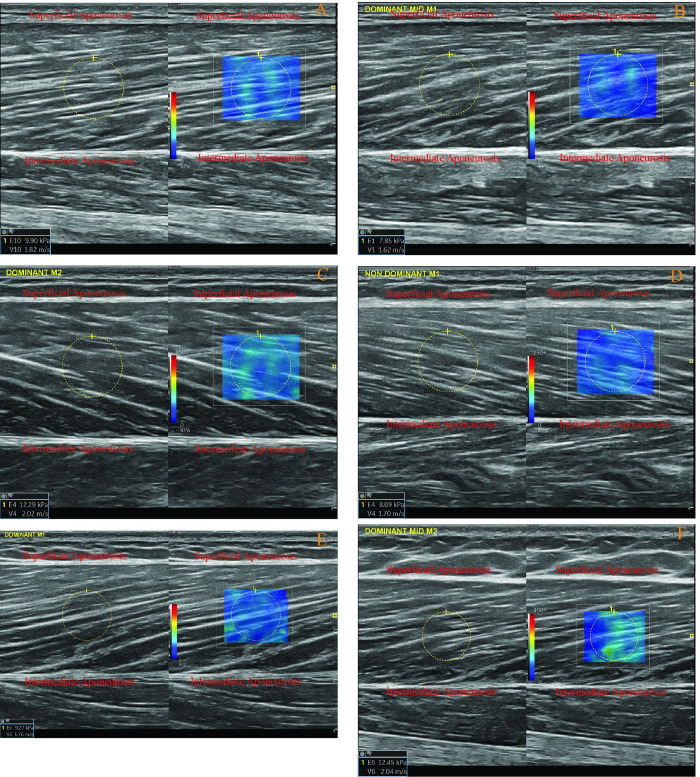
A-F: Random examples of the measurements of mid-muscle belly passive stiffness of the biceps femoris long head via ultrasound-based shear-wave elastography in various participants. The different image orientations, whether from left to right or vice versa, result from the 180° differences in the grip positions of the ultrasound probes during each measurement, which do not affect the outcomes.
